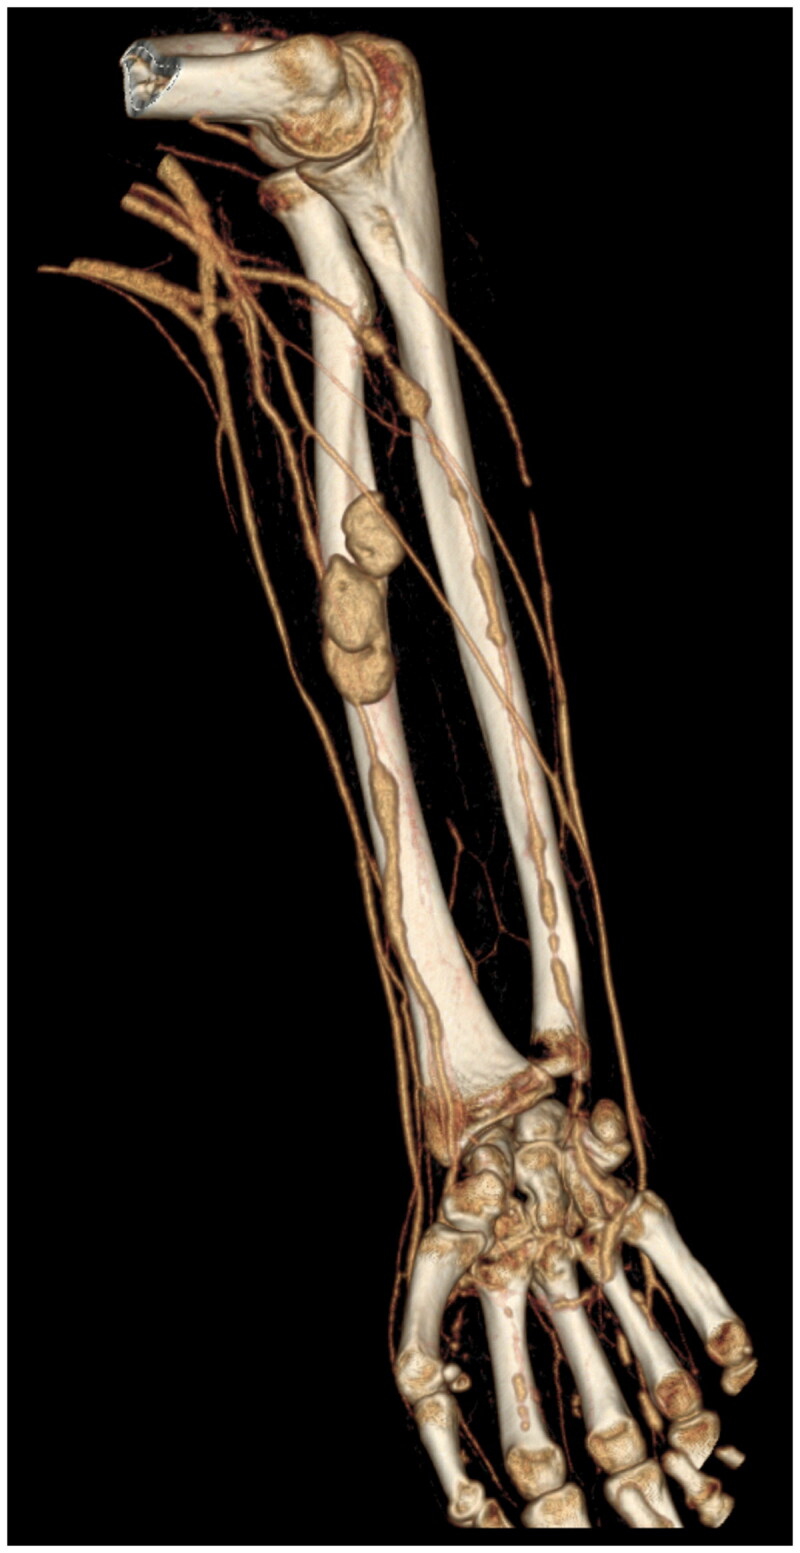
CT Angiogram showed multiple pseudoaneurysms along radial and ulnar arteries.
